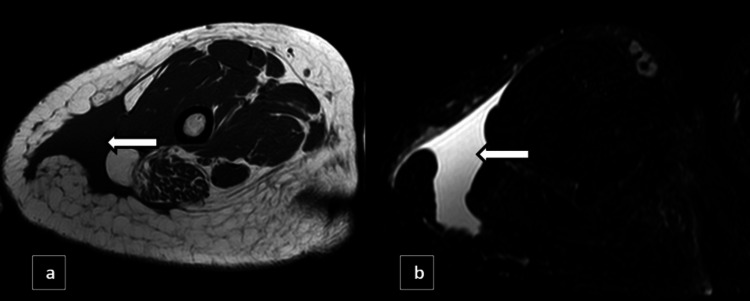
MRI T1 axial reformatted image (a) and MRI short tau inversion recovery (STIR) axial reformatted image (b) showing a fairly defined T1 hypointense and STIR hyperintense fluid collection noted between the subcutaneous and muscle layers of the right lateral aspect of the thigh region (white arrows).
